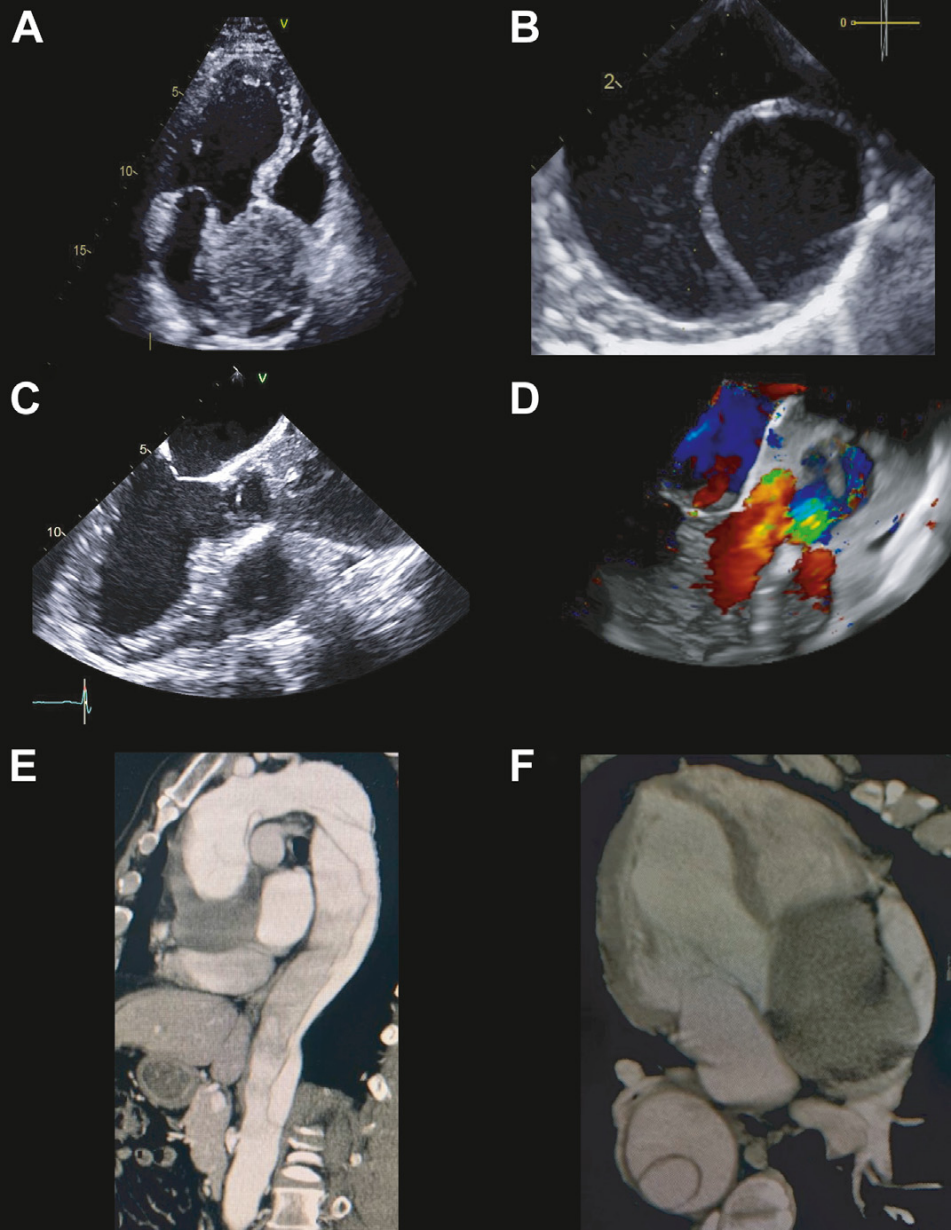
Multimodality Imaging of Massive Thrombus in Chronic DeBakey I, Stanford Type A Aortic Dissection (A) Presurgery transthoracic echocardiogram, in the apical long-axis view, shows the thrombus in the aortic root. (B) Transesophageal echocardiogram demonstrates the thickness of the dissection flap in the thoracic aorta, thus denoting chronicity. (C and D) Transesophageal echocardiogram in 2 and 3 dimensions confirms the documented findings, and it also reveals an ascending thoracic aortic aneurysm measuring 6.6 cm and severe aortic regurgitation that is caused by a mixed mechanism: aortic dilatation and thrombus occupation of the aortic root. (E and F) Computed tomography reaffirms the findings and establishes the extent of the dissection.
